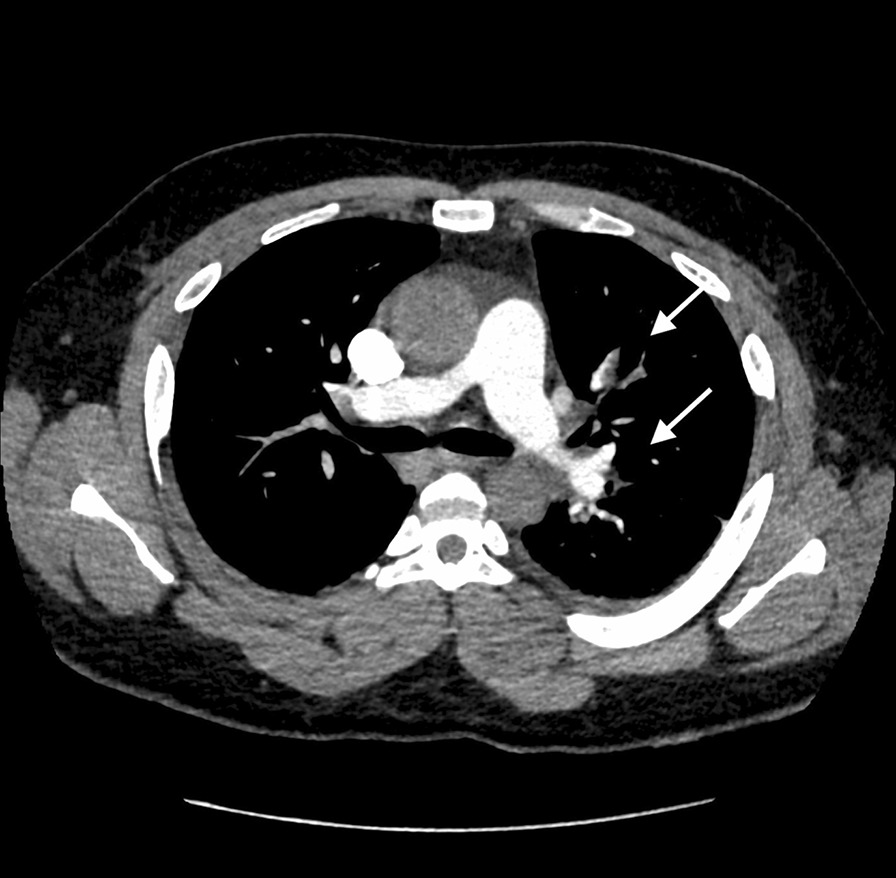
Computed tomography (CT) pulmonary angiogram shows distal pulmonary embolisms in inferior lingular segment at the upper lobe, lateral and posterior segments in lower lobe
